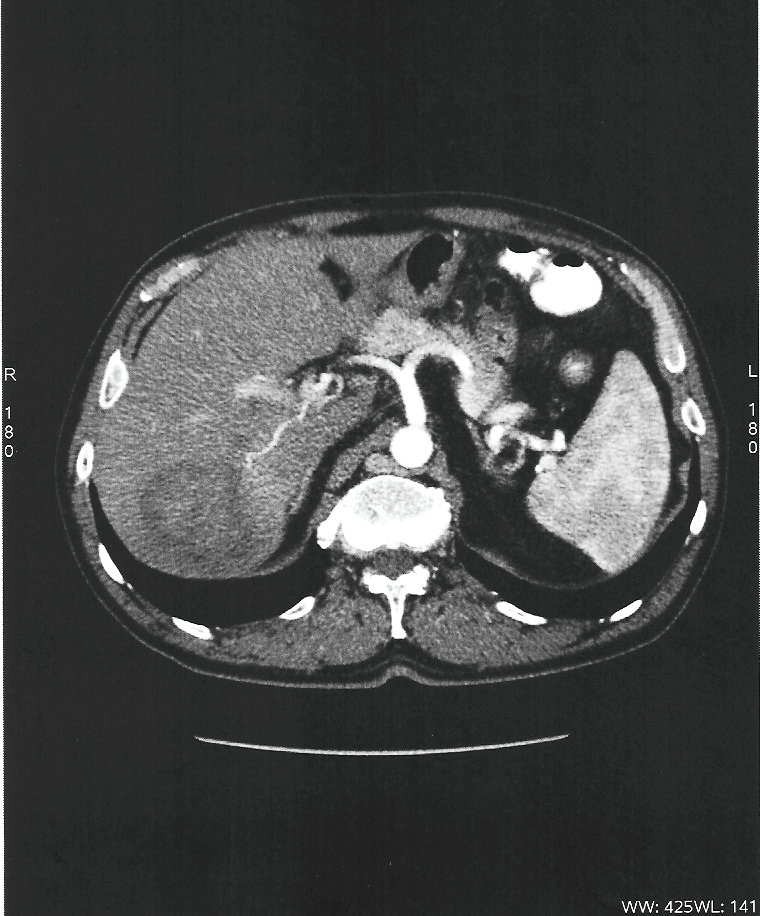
CT scan showing a well-encapsulated round tumor, with low density and low enhancement of the tumor during the arterial phase.
